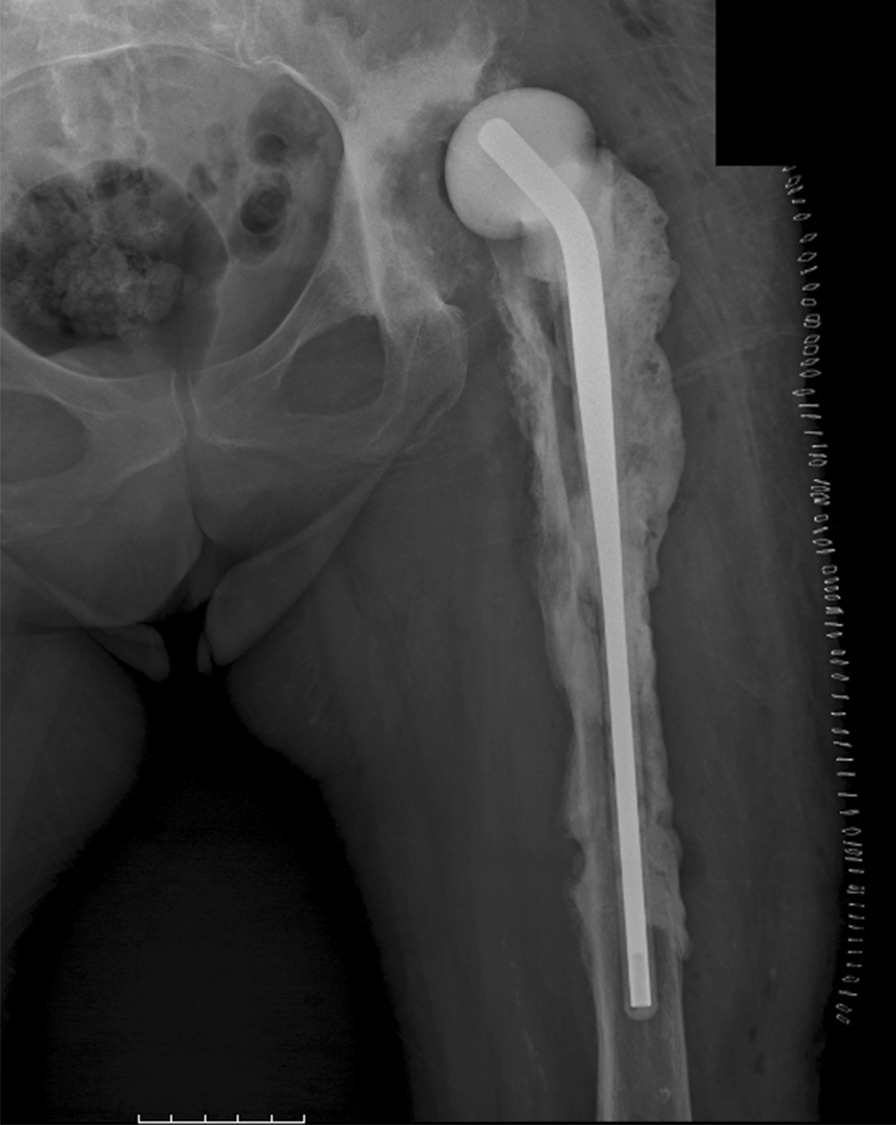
Case no. 20, X-rays showing a subluxated spacer in situ
